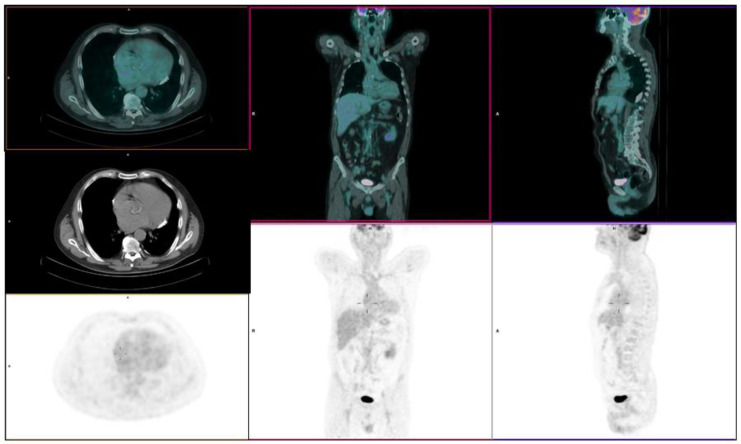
June 2020; 18F-FDG PET/CT after 7 cycles of chemotherapy (R-COMP) showing an early complete response to treatment.
